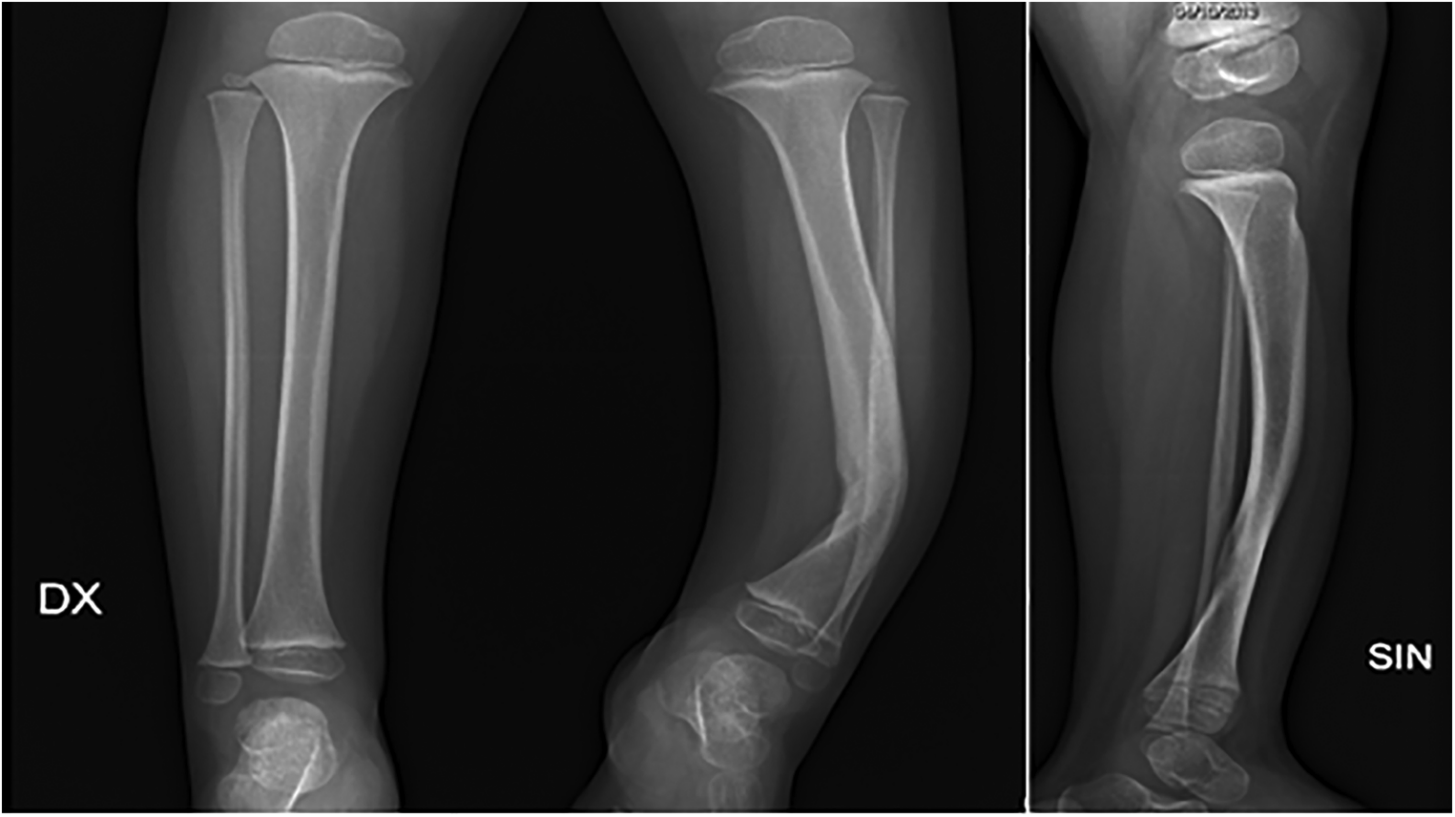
Initial x-rays (AP and LL view) of the anterolateral congenital tibial curvature.
